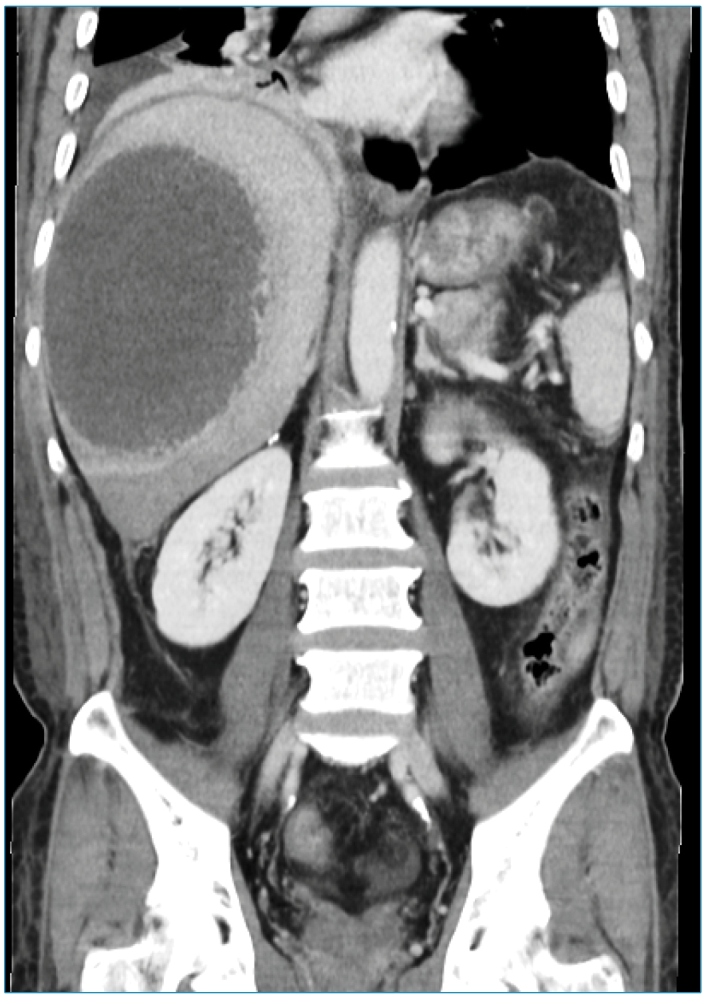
Computed tomography of the abdomen showing a large non-enhancing hypodense liver lesion, suggestive of a liver abscess.
